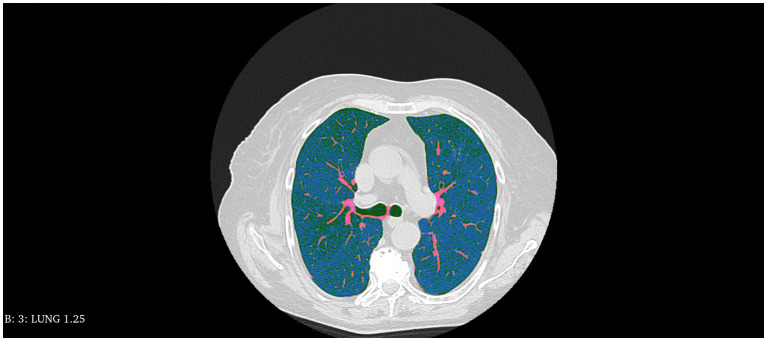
Exploratory attenuation-based aeration mapping on follow-up inspiratory CT scan. Segmentation was performed using 3D Slicer (version 5.8.1) based on predefined attenuation thresholds. Hyperinflated regions (−1,000 to −901 HU; shown in dark green) are identified in the anterior segment of the right upper-lobe, the middle lobe, and the left upper-lobe (culmen). Normally aerated lung regions (−900 to −501 HU; shown in blue) largely correspond to areas that showed consolidation on CT performed 3 months earlier.
